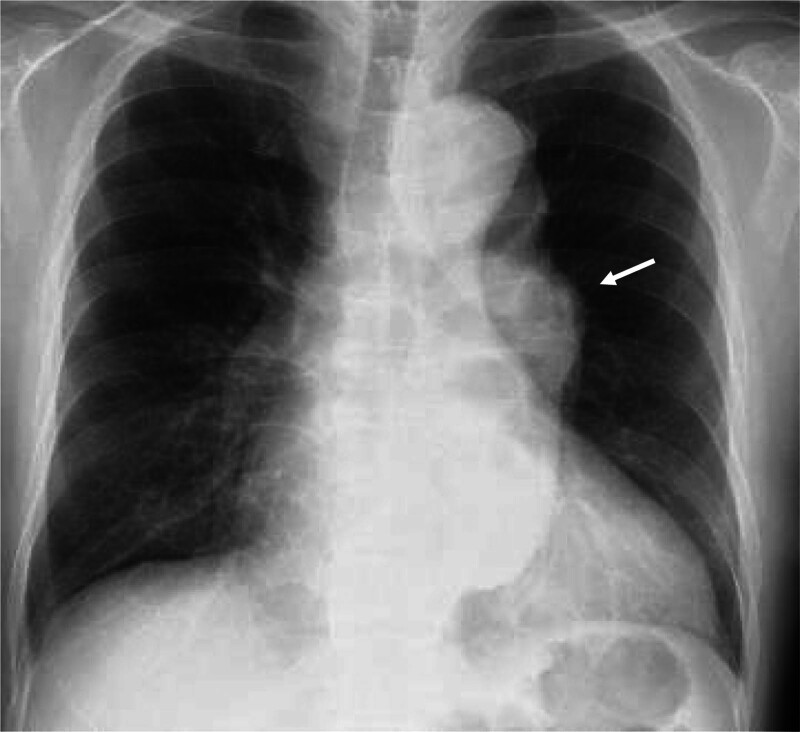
Preoperative chest radiography findings. Chest radiography indicated a cardiothoracic ratio of 65%. The white arrow indicates descending aortic aneurysm.
